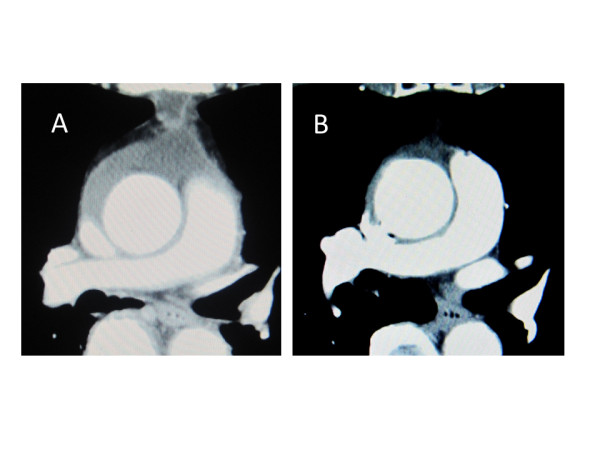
Postoperative computed tomography.A. Two weeks after the operation, perigraft fluid collection was detected. B. Two years after the operation, perigraft fluid collection was disappeared.
